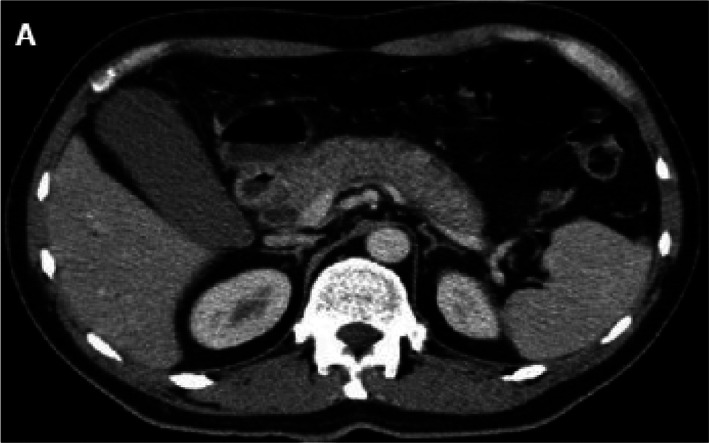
Contrast‐enhanced CT reveals diffuse pancreatic enlargement (i.e. sausage pancreas) with “featureless borders” and a capsule‐like rim, which is characteristic of AIP.^46^
